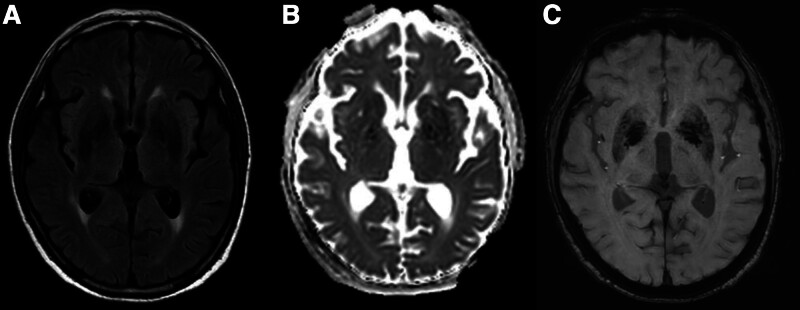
Follow-up brain MRI at 5 weeks after admission. (A–C) FLAIR, ADC, and SWI images demonstrate resolution of the previously noted bilateral globus pallidus lesions. ADC = apparent diffusion coefficient, FLAIR = fluid attenuation inversion recovery, SWI = susceptibility weighted imaging.
